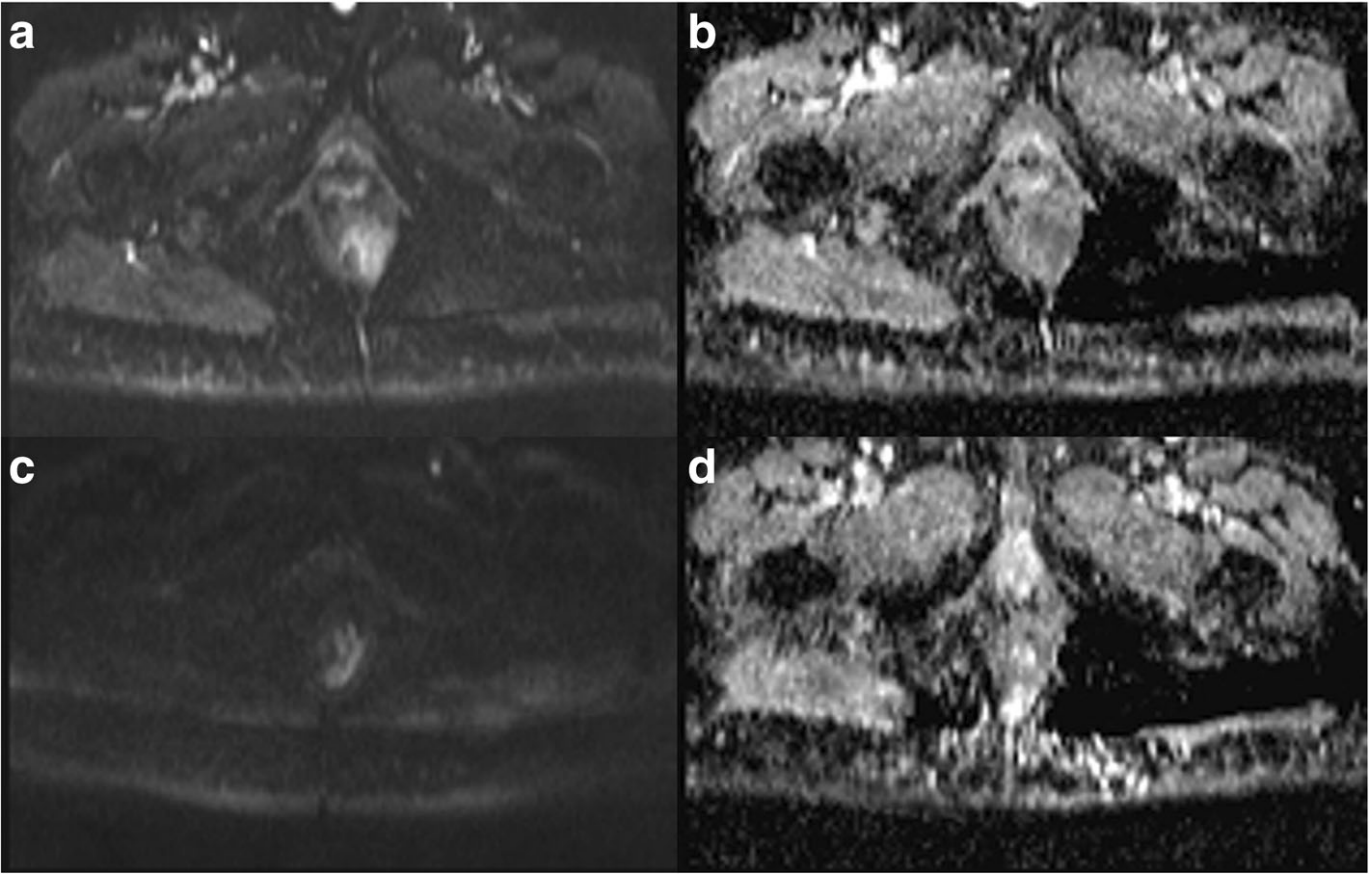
Same patient of 4 and 5: DWI sequences. In (a) b800 pre-treatment examination: cancer shows hyperintese signal, in (b, a, d, c): the lesion appear hypointhense. In (c) post treatment b800, a lower signal than in A with a higher signal in (a, d, c) than in b
